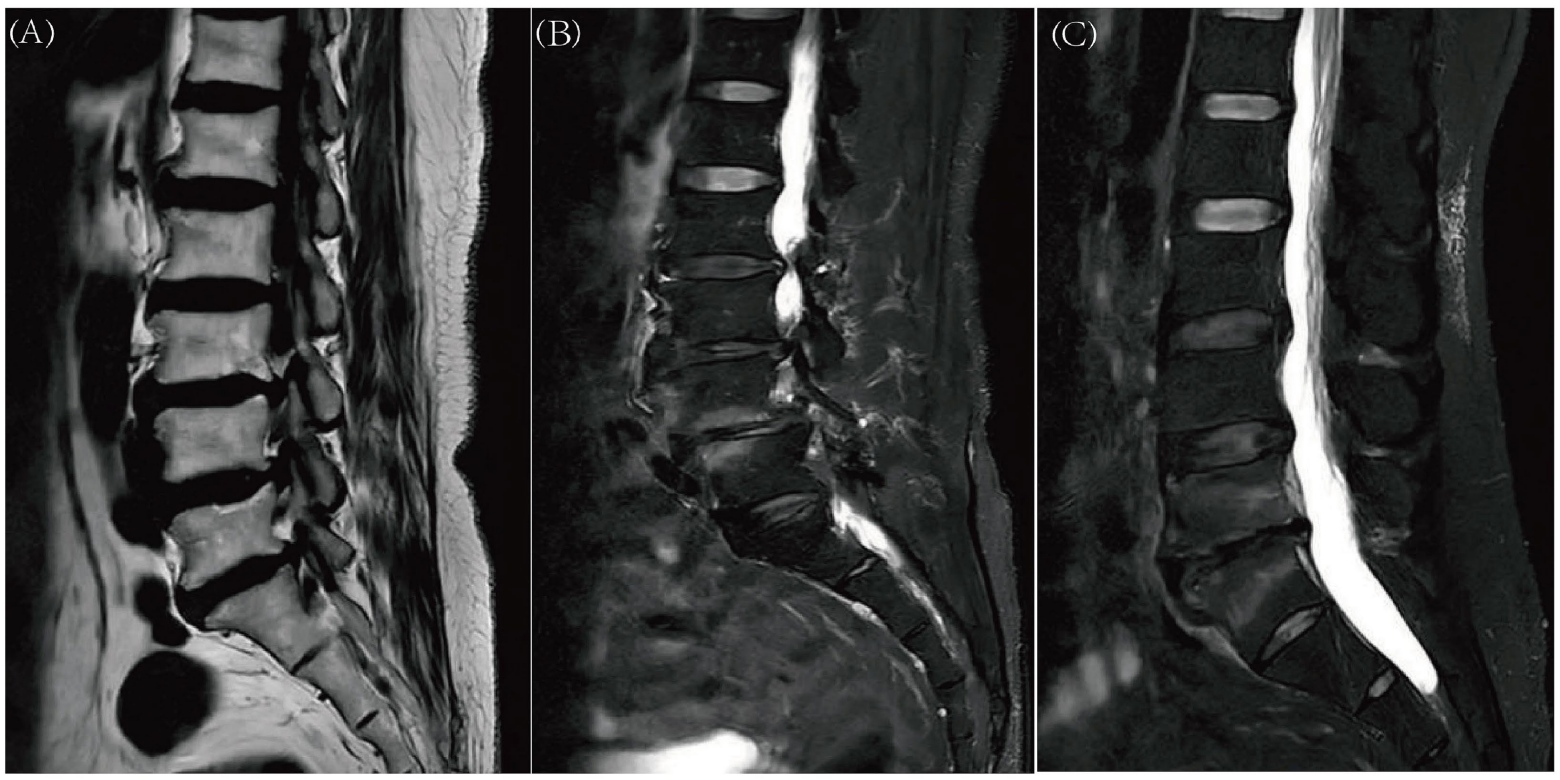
Grade of Modic changes Grade A: marrow changes of <25% of the vertebral height (A). Grade B: marrow changes between 25 and 50% of the vertebral height (B). Grade C: marrow changes of >50% of the vertebral height (C). Classification of Modic changes type I: signal hyperintensity is also observed on fat-suppressed T2-weighted MRI (B,C). Classification of Modic changes type II: signal hyperintensity is identified on T1-weighted MRI (A).
